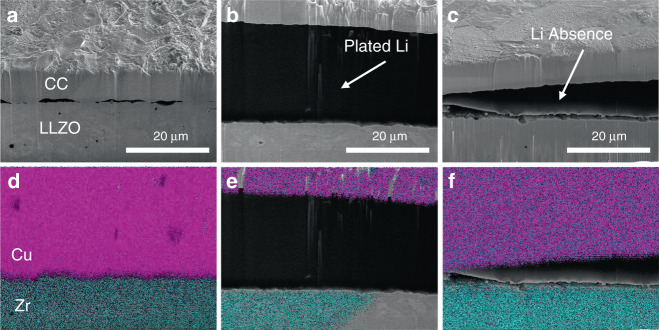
Cross-sectional SEM at the interface of the LLZO and the current collector. SEM (a) as assembled, (b) after plating 5 mAh cm^−2^ of Li, and (c) after plating and then stripping of 5 mAh cm^−2^ of Li. Elemental maps for Cu and Zr at the interface (d) as assembled, (e) after plating, and (f) after plating and stripping. Metallic Li is observed under secondary electrons in between the Cu and LLZO layer in b but cannot be detected since the characteristic X-ray energy falls outside of the detection range of EDS.
